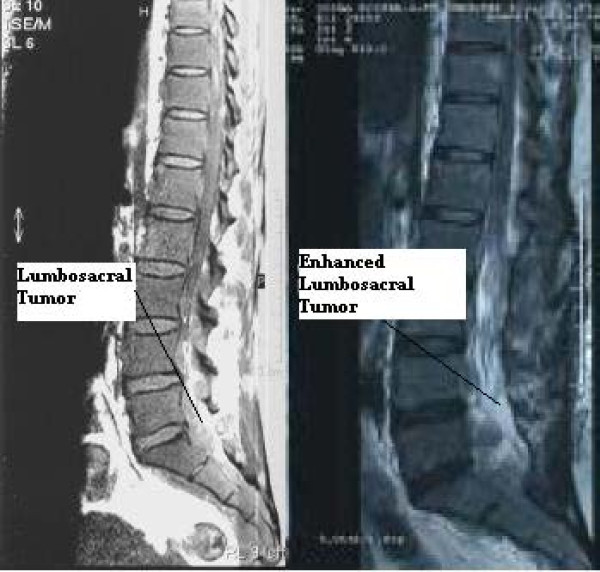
An MRI of the lumbosacral spine (left) showed an extradural lesion extending from L3 to S3 (left), which became enhanced after a contrast injection (right).
